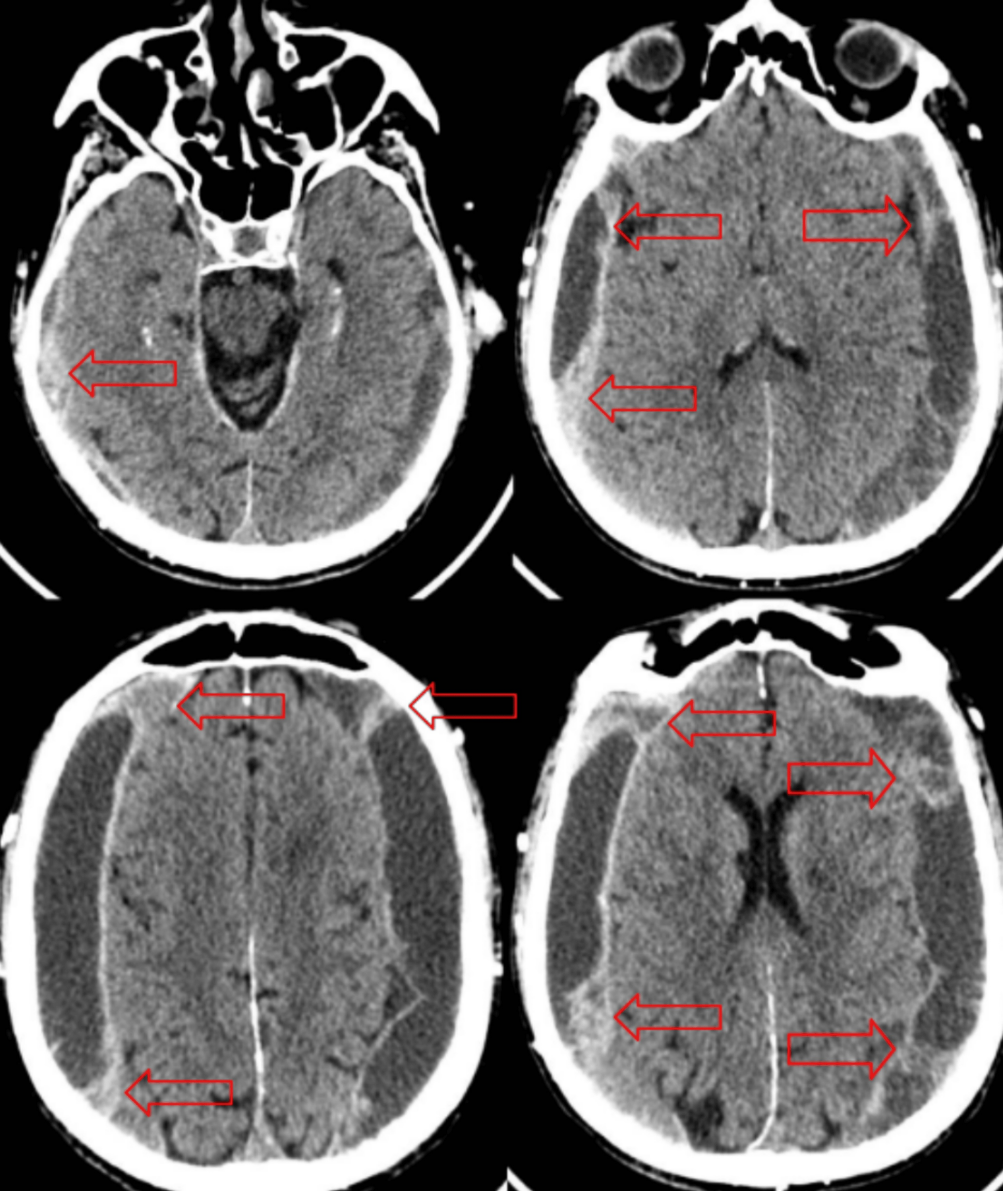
Several slides of the initial computed tomography scan of the head depicting acute on chronic subdural hematomas (red arrows) with mass effect on the adjacent brain parenchyma and bilateral lateral ventricles. There was no evidence of a significant midline shift or large acute territorial infarct.
